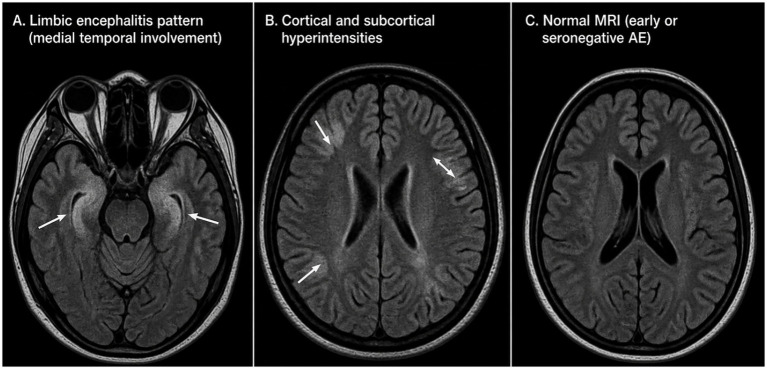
Representative MRI findings in autoimmune encephalitis. (A) Limbic encephalitis pattern demonstrating bilateral mesial temporal (hippocampal) hyperintensities on axial FLAIR imaging (arrows). (B) Non-limbic involvement with patchy cortical and subcortical hyperintensities in extra-temporal regions (arrows), suggesting diffuse neuroinflammatory alterations. (C) Normal-appearing MRI without focal signal abnormality. Normal imaging does not rule autoimmune encephalitis and is frequently seen in early-stage illness or anti-NMDAR encephalitis.
